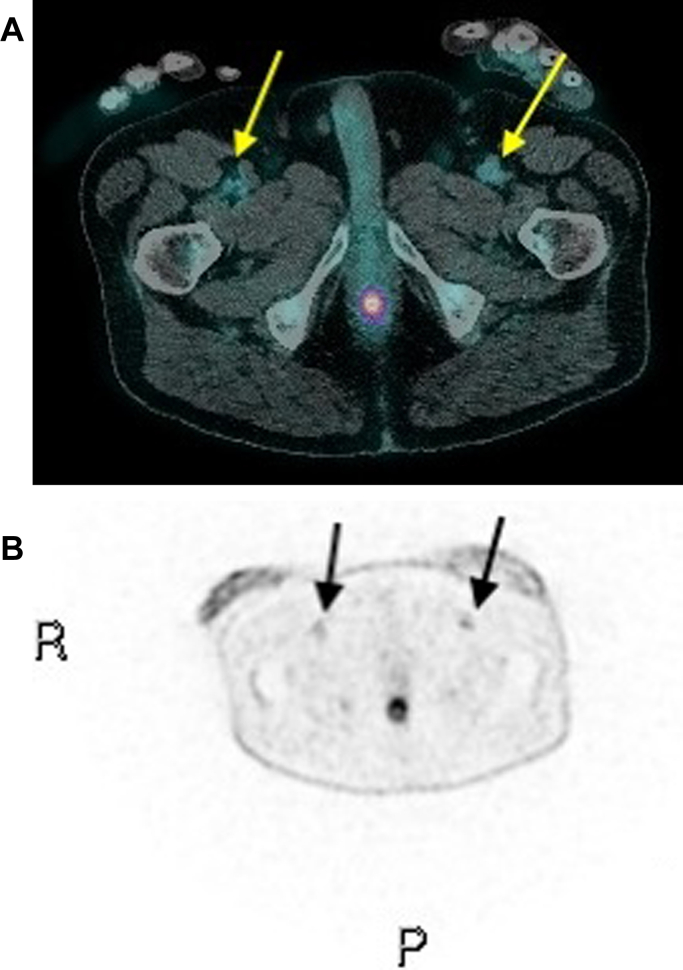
Fluorodeoxyglucose-Positron Emission Tomography Scan Whole-body fluorodeoxyglucose-positron emission tomography (FDG-PET) scan performed on day 21; fused (A) and native (B) images showing enhancement of bilateral femoral arteries (yellow arrows, black arrows), indicative of medium-vessel vasculitis. P = posterior; R = right.
